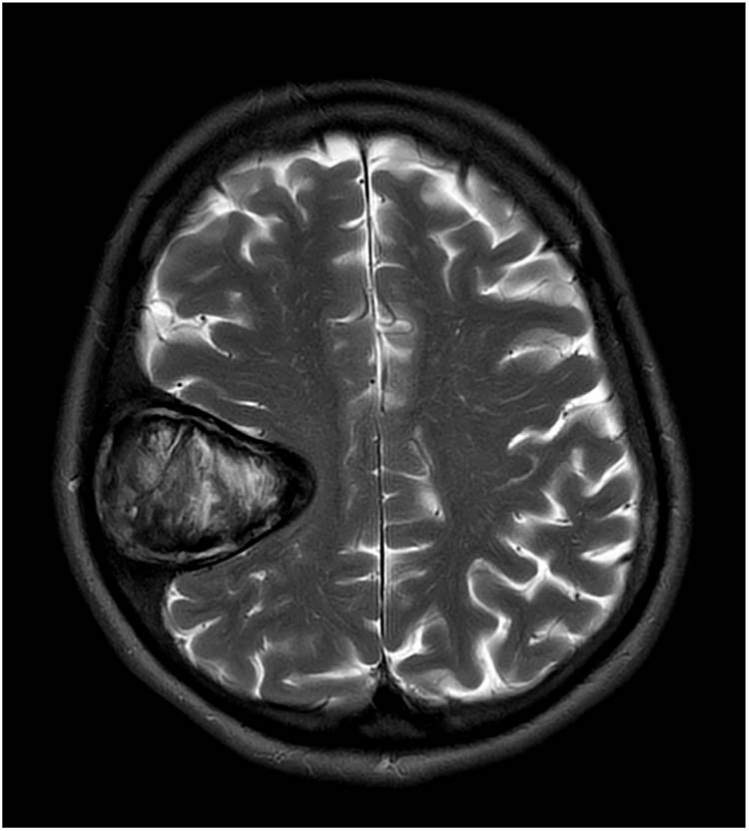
T2-weighted axial MRI scan showing the lesion with intralesional haemorrhagic products. There is no evidence of dural invasion.
